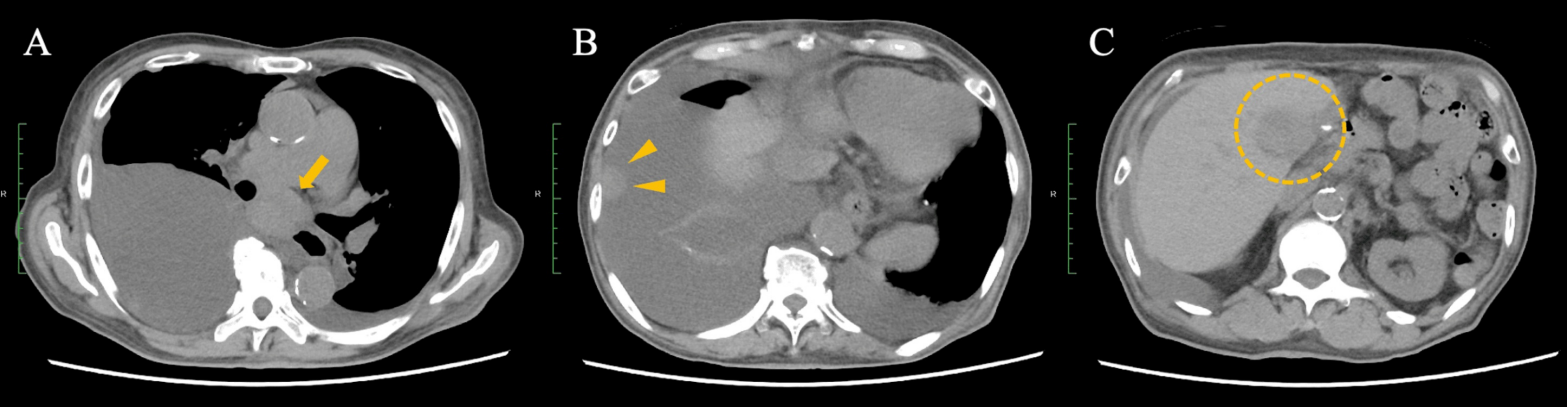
CT images obtained after two cycles of treatment with durvalumab plus tremelimumab (A) Chest CT shows enlargement of mediastinal lymph nodes with a new, large right pleural effusion (arrow). (B) Pleural dissemination is observed within the right pleural effusion (arrowheads). (C) Abdominal CT demonstrates interval enlargement of intrahepatic metastatic lesions (dotted circle). These findings warranted classification as progressive disease on durvalumab plus tremelimumab.
